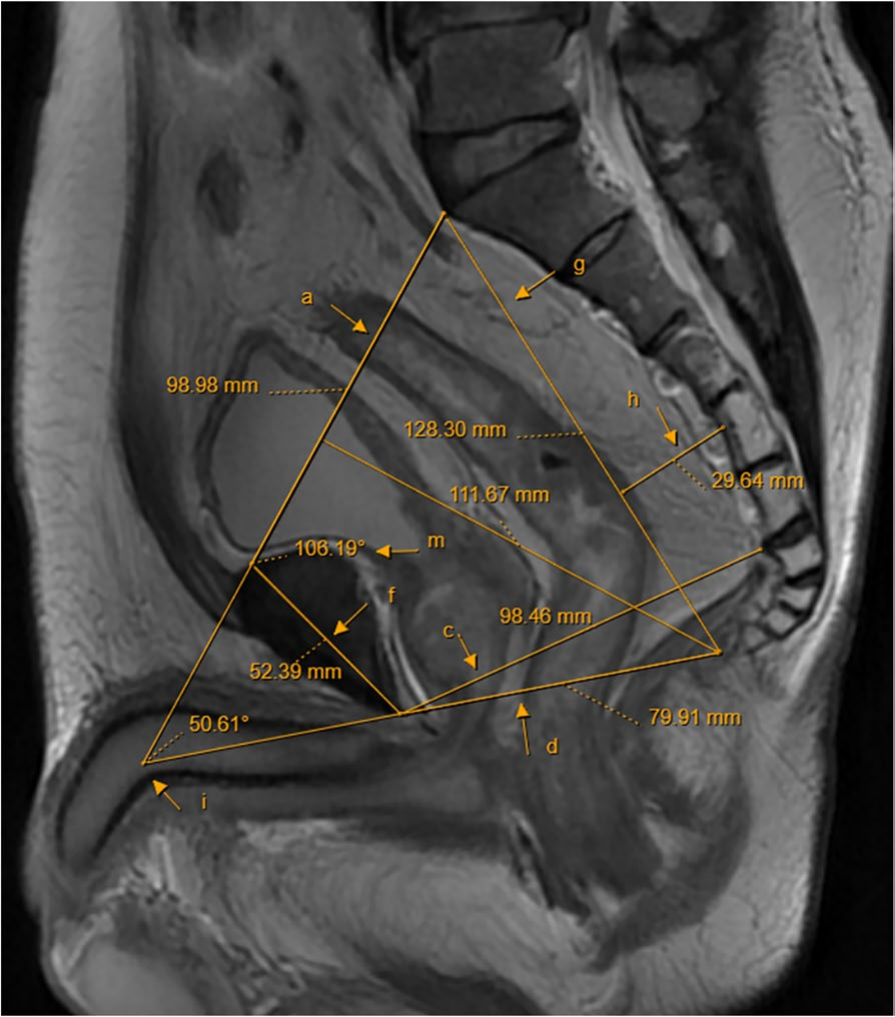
Sagittal pelvic magnetic resonance images: a anteroposterior diameter of the pelvic entrance, c anteroposterior diameter of the middle pelvis, d anteroposterior diameter of the pelvic outlet, f pubic symphysis height, g sacrococcygeal distance, h sacrococcygeal curvature depth, i sacrococcygeal–pubic angle, j pelvic depth, and m Angle A5
